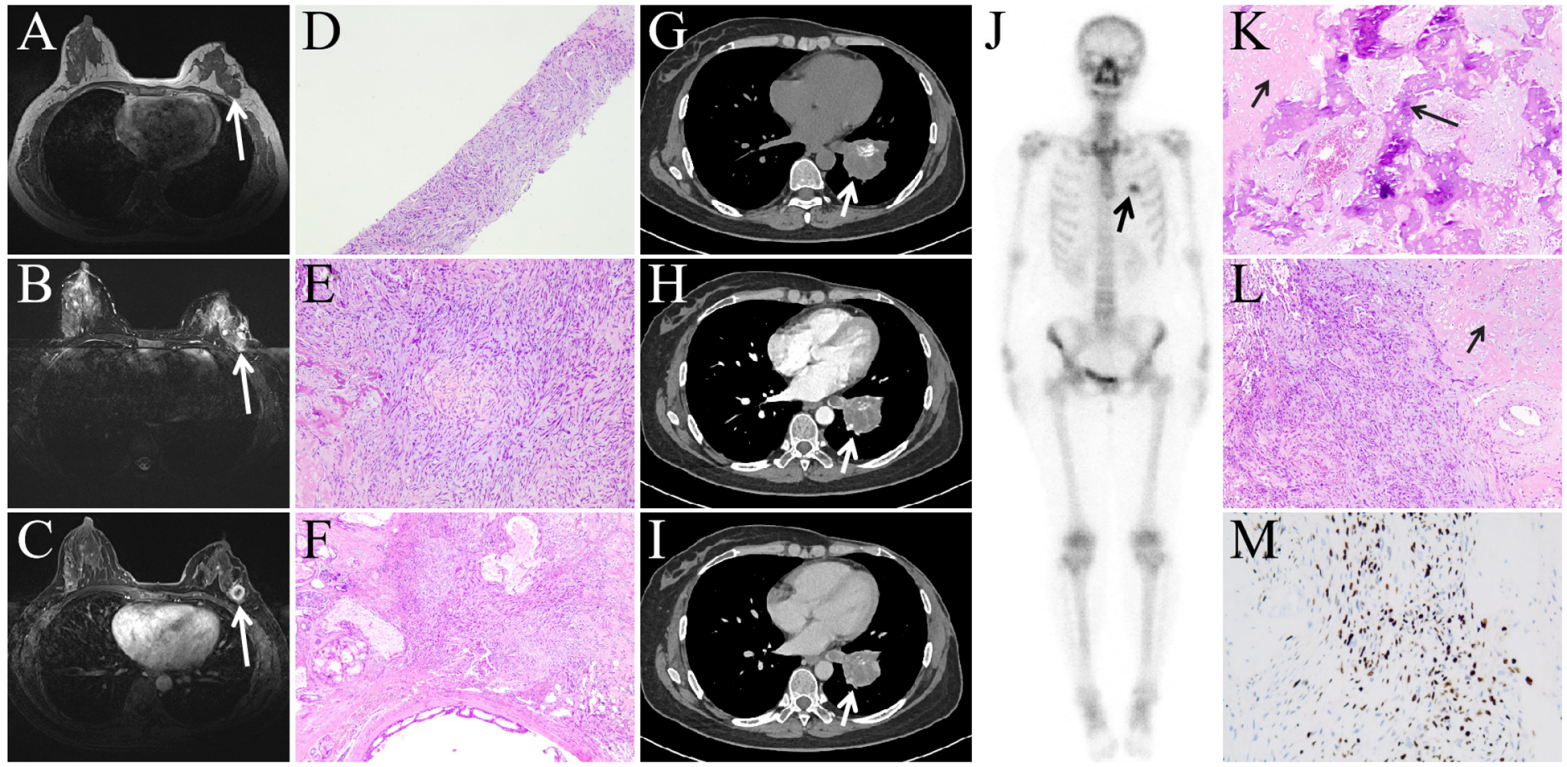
Breast MRI (A: T1WI, B: T2WI, C: contrast-enhanced scan) revealed a nodule in the left upper outer quadrant (long arrow), exhibiting long T1WI and long T2WI signals, with ring enhancement on contrast-enhanced scan. The biopsy result of the breast nodule (D: HE, 4×10, E: HE, 20×10, F: HE, 10×10) showed numerous spindle cells with heterologous components of bone and cartilage. Contrast-enhanced CT examination (G-I) revealed a mass in the left lower lobe (short arrow), with mild enhancement on contrast-enhanced scan, patchy high-density areas were also observed within the mass, which demonstrated intense radiotracer uptake on whole-body bone scintigraphy images (J, long arrow),suggesting active bone metabolism within the mass, consistent with the presence of bone and cartilage components found in the postoperative pathological images. Figures K-M (K: HE, 10×10, L: HE, 10×10, M: DAB, 10×10) represent postoperative pathological images of the left lower lobe lung lesion, which, similar to the breast lesion case results, also revealed heterologous components of bone (K, long arrow) and cartilage (K-L, short arrow).
